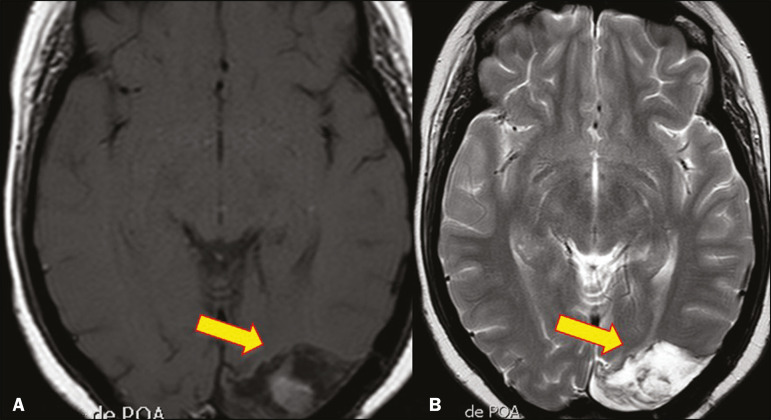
A 20-year-old patient with angioinvasive aspergillosis of the CNS. A: Axial T1-weighted MRI sequence showing a hyperintense aspergilloma, with a hypointense halo, in the left occipital region (arrow). B: Axial T2-weighted sequence showing a hyperintense lesion (arrow).
